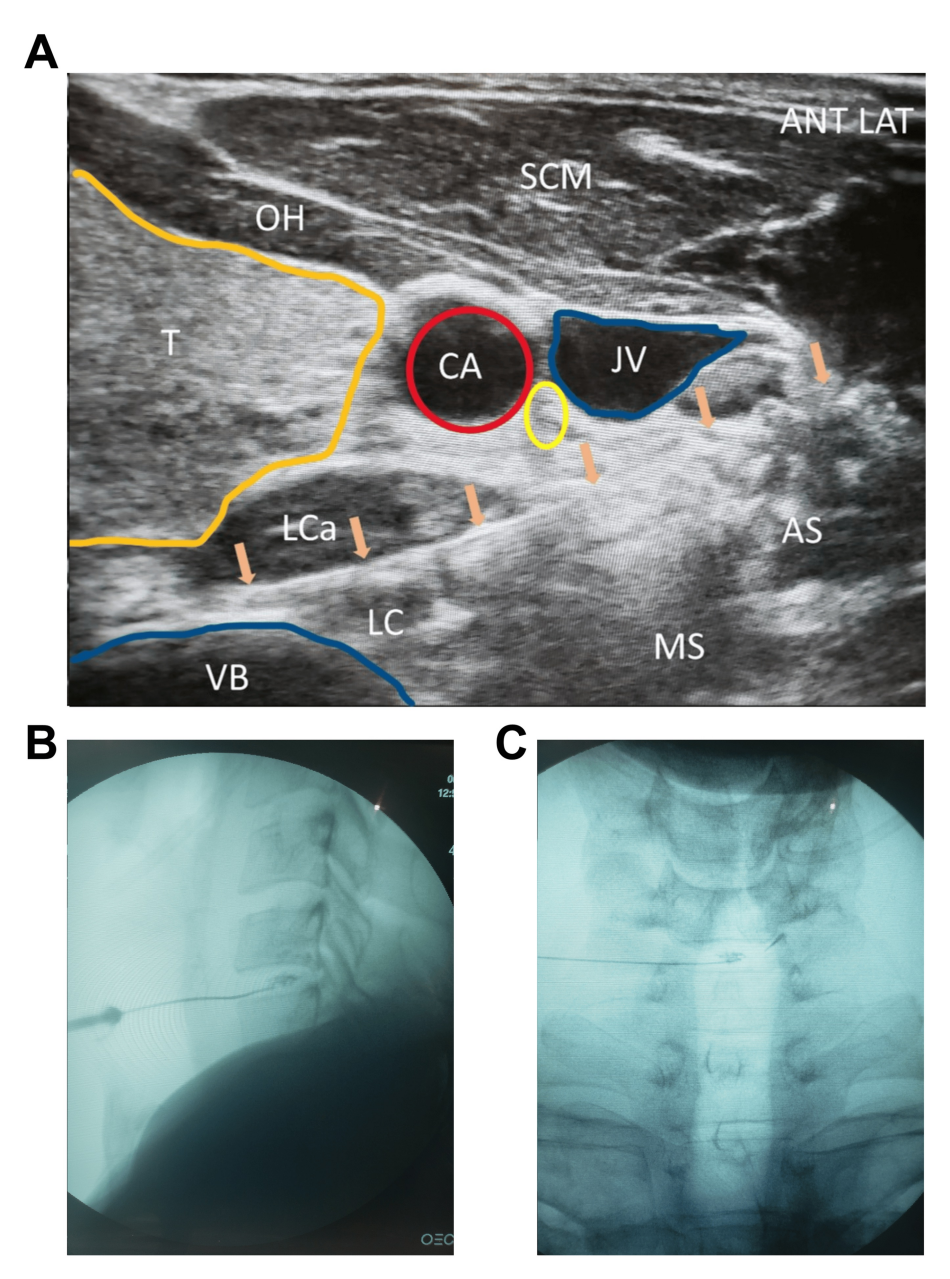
Cervical Intradiscal Injection Technique. Needle advanced deep to the great vessels under linear in-plane ultrasound guidance. Arrows indicate needles. Yellow circle denotes vagus nerve. ANT LAT, anterior lateral; T, thyroid; CA, carotid artery; JV, jugular vein; VB, cervical vertebral body; LC, longus colli; LCa, longus capitis; AS, anterior scalene; MS, middle scalene; OH, omohyoid.
